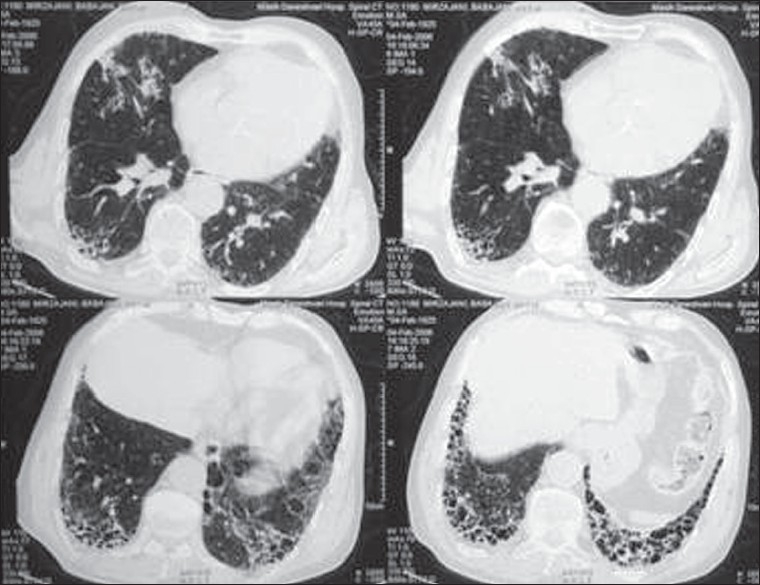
CT scans in usual interstitial pneumonia
